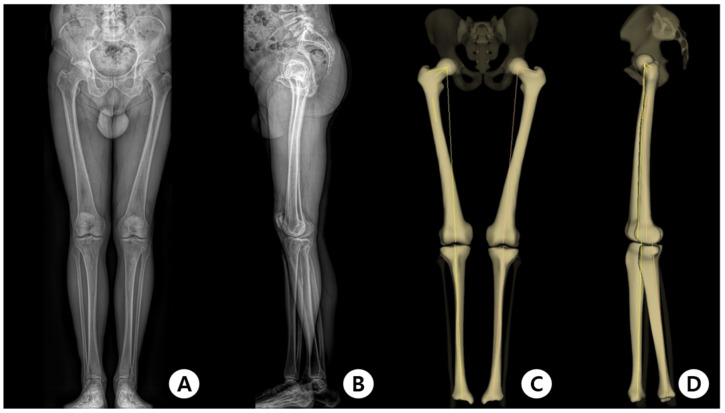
(A,B) Anteroposterior and lateral radiographs acquired for 3D reconstruction; (C,D) The 3D reconstruction images made by the EOS imaging system.
